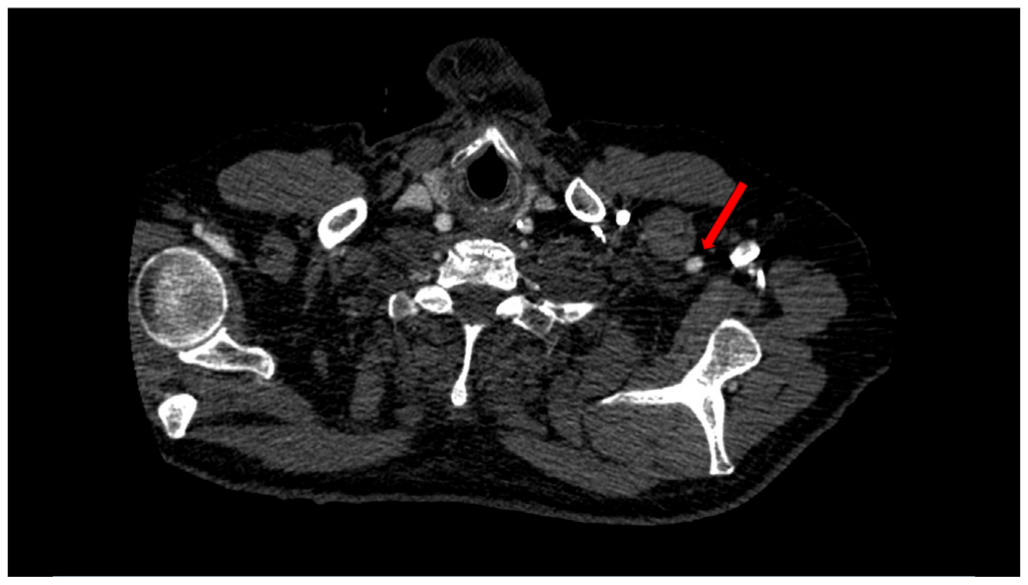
Computed tomographic angiography (CTA) reveals a left axillary artery suitable to advance the endovascular stent draft during endovascular aortic aneurysm repair (EVAR).
